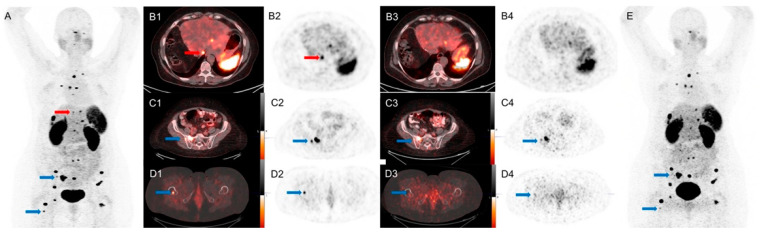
A 65-year-old woman with metastatic NET of unknown primary, referred for subsequent treatment strategy after chemotherapy. SiPM-based PET/CT was performed first at 72 min after injection of 4.2 mCi of ^68^Ga-DOTA-TATE. Conventional PET/CT was performed second at 96 min after injection. Red arrows mark an additional lesion seen in the dome of the liver only on SiPM-based PET/CT (A,B1), not on conventional PET/CT (B3,B4,E). Blue arrows mark bone lesions seen on conventional PET/CT (C3,C4,D3,D4,E) but more conspicuous on SiPM-based PET/CT (A,C1,C2,D1,D2).
